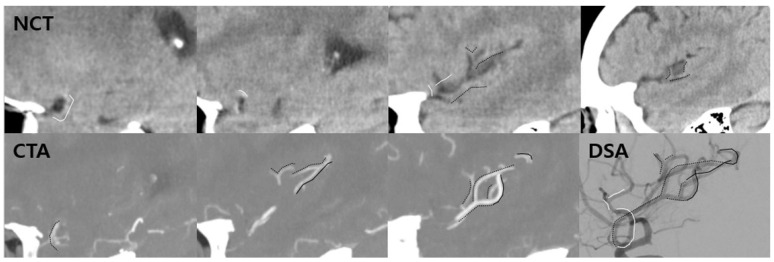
Upscaling of arterial visualization grade after comprehensive reading. A patient with MCA occlusion shows NCT grade 3 of distal-to-thrombus segment and CTA grade 3 of distal-to-thrombus segment. After comprehensive reading, the arterial visualization grade of the distal-to-thrombus segment was 5. (NCT) A small portion of the inferior division and superior division of MCA was visible on NCT. (CTA) Almost all the portion of the inferior division and no superior division was visible on CTA. (DSA) Piecing arterial course together from NCT and CTA constitutes the entire MCA branching pattern. White line = arterial course visualized on NCT only; black dotted line = visualized both on NCT and CTA; black solid line = visualized on CTA only. MCA, middle cerebral artery; NCT, non-contrast computed tomography; CTA, computed tomography angiography; DSA, digital subtraction angiography.
